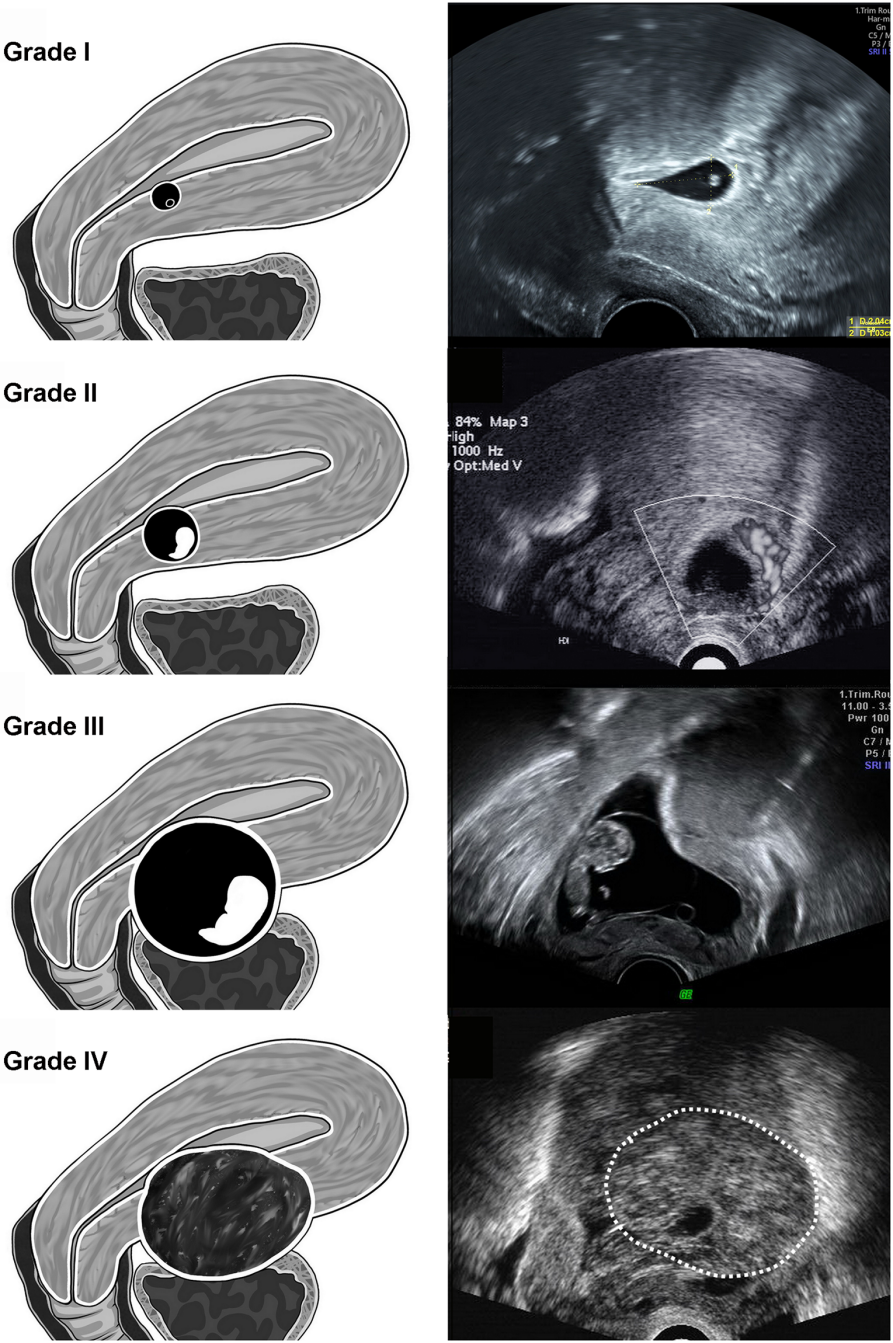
The description of our new ultrasound grading system for CSP. Grade I CSP represented the depth of CSP embedded in less than one-half thickness of the lower anterior corpus. Grade II CSP implied CSP occupied more than one-half thickness of the lower anterior corpus. In grade III CSP, the GS bulged out the overlying myometrium and uterine serosa. In grade IV CSP, the GS became an amorphous tumor with rich vascularity at the cesarean scar.
